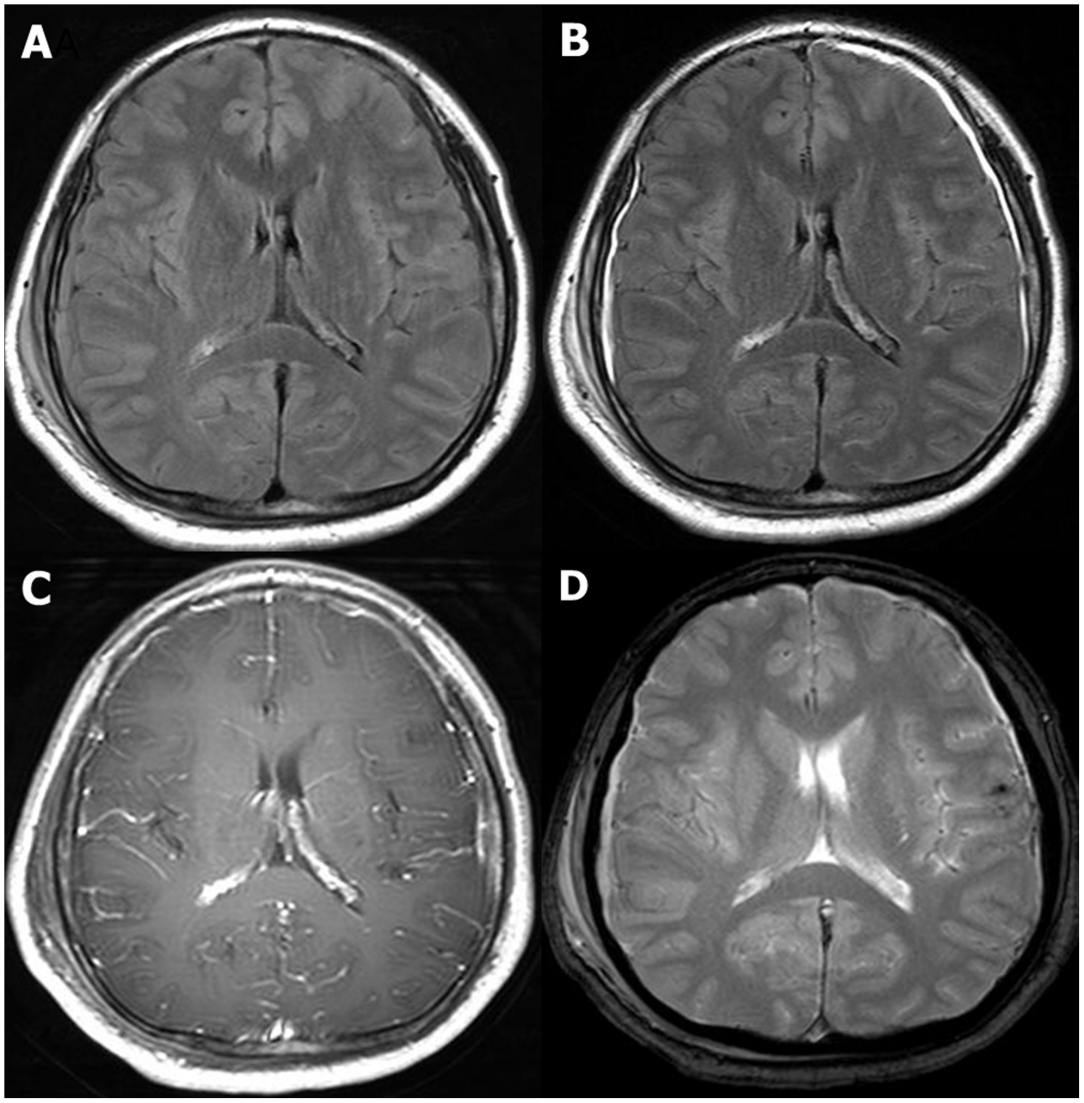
A 67-year- old male patient with a history of fall down 4 hours ago. Initial GCS score was 13. The duration of posttraumatic amnesia was 1(A) Unenhanced FLAIR MR image shows small amount of subdural hemorrhage with iso-signal intensity in Rt. parietal convexity. (B) contrast-enhanced FLAIR MR image clearly demonstrates meningeal enhancement along not only right convexity but also Lt. side. (C) contrast-enhanced T1 weighted image shows no definite enhancement. (D) GRE image depicts hemosiderin deposition only in Lt. cerebral cortex.
